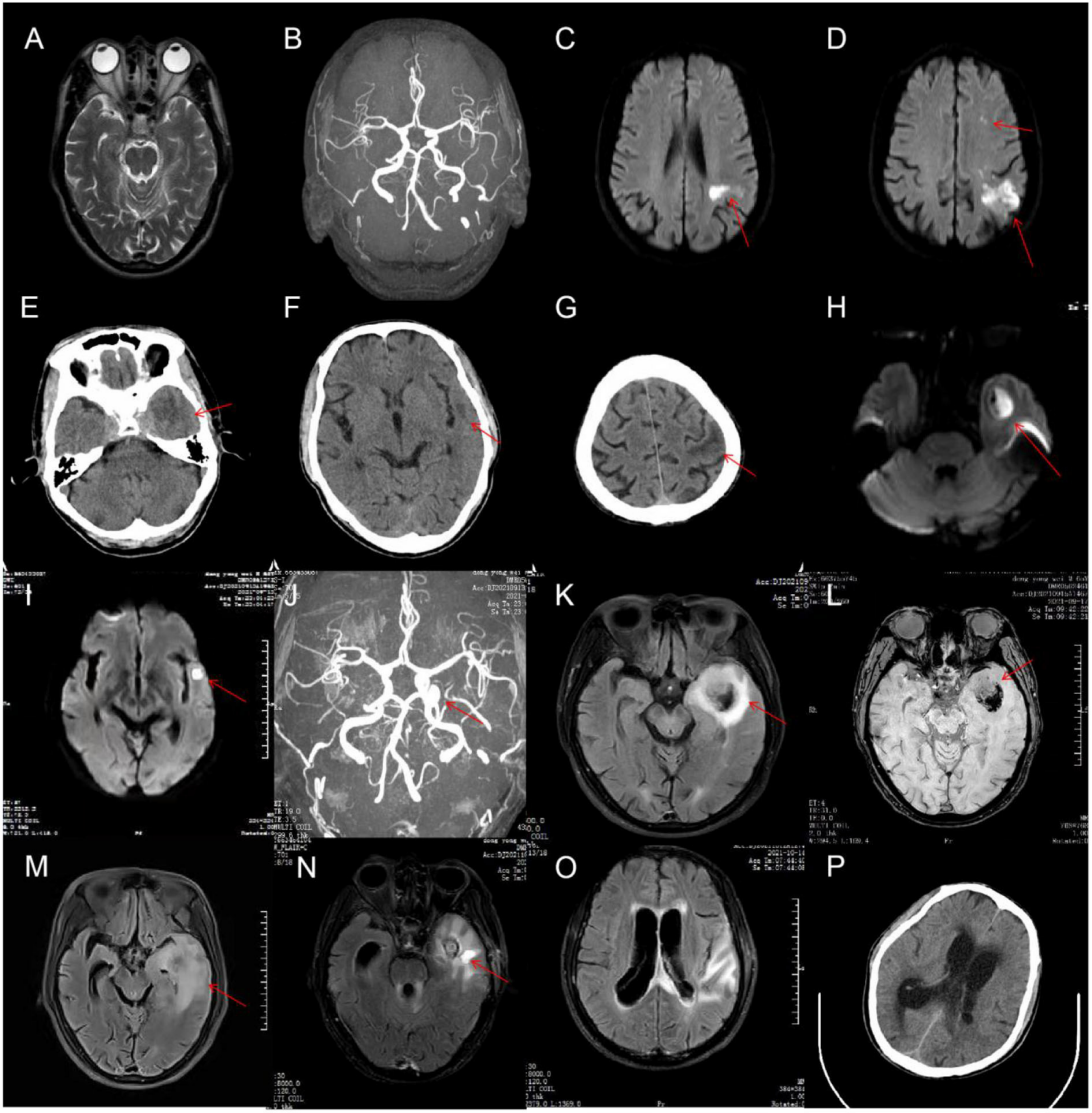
On admission, cranial magnetic resonance imaging (MRI) of the patient was normal (A), and there was no evidence of intracranial aneurysm on brain magnetic resonance angiography (MRA) (B). On hospital day 7, a brain MRI was done again and showed acute multiple cerebral infarcts in the left cerebral hemisphere (C,D). On hospital day 25, cranial CT revealed significantly increased low-density shadow in the left frontal, temporal, and parietal lobes (E–G). The MRI (H,I) and MRA (J) re-examination demonstrated that the lesions in the left cerebral hemisphere were increased significantly, and a cerebral aneurysm formed in the intracavernous segment of the left internal carotid artery (ICA). On hospital day 29, a repeat head MRI showed markedly enlarged size of the left temporal lesions with abscess formation and bleeding (K,L). On hospital day 37, brain MRI showed a decreased size of the left temporal lesions but with perifocal evident edema (M). On hospital day 55, a repeat head MRI showed that the lesions and edema of the left temporal lobe was improved, but hydrocephalus became even worse (N,O). On hospital day 58, cranial CT showed intraventricular extension and hydrocephalus (P).
